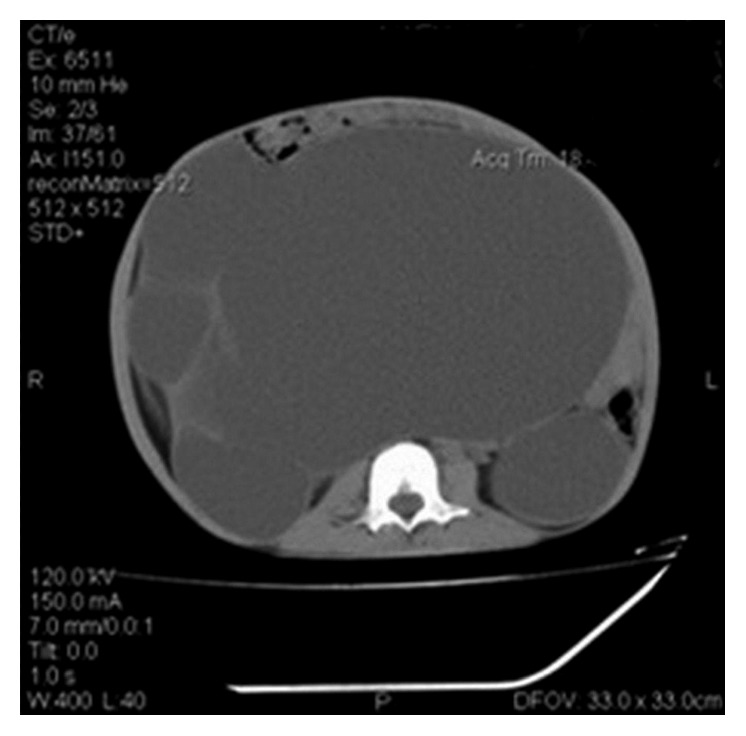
CT abdomen showing the renal mass occupying the entire abdomen.
